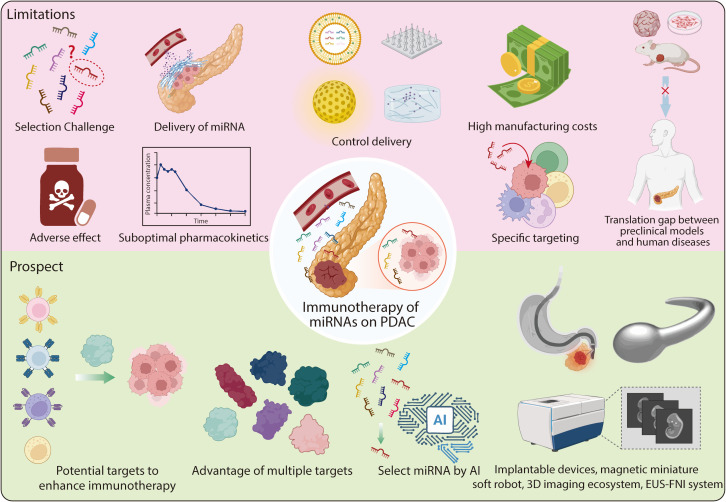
The limitations and prospect for immunotherapy of microRNAs on PDAC. The limitations of miRNA-based immunotherapy for PDAC include the following points: First, it is difficult to identify the most efficient miRNA for immunotherapy in PDAC. Second, adverse effects. Third, delivery of miRNA. Fourth, controlling the delivery of miRNA. Fifth, achieving precise targeting of specific cells or places. Sixth, translation gap between preclinical models and human diseases. Seventh, suboptimal pharmacokinetics of miRNAs. Eighth, high manufacturing costs of miRNA. The prospect of miRNA-based immunotherapy for PDAC includes: First, potential targets to enhance the efficiency of immunotherapy in PDAC. Second, miRNA can target multiple molecules to achieve regulation of multiple molecules and pathways. Third, the application of technologies like implantable devices, EUS-FNI system, magnetic miniature soft robots and more precise 3D imaging ecosystems to improve immunotherapy. Fourth, selecting miRNAs using AI to improve efficiency. EUS-FNI, endoscopic ultrasound-guided fine needle injection; AI, artificial intelligence.
